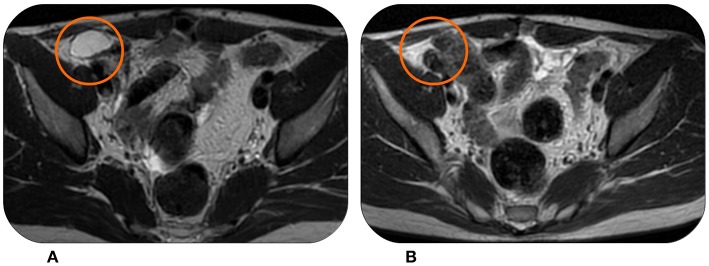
This patient experienced in June 2016 disease progression with multiple peritoneal lesions at MRI (A) after being treated with Trabectedin and surgery. However, Trabectedin showed to be effective again, achieving a partial response in February 2018 (B).
